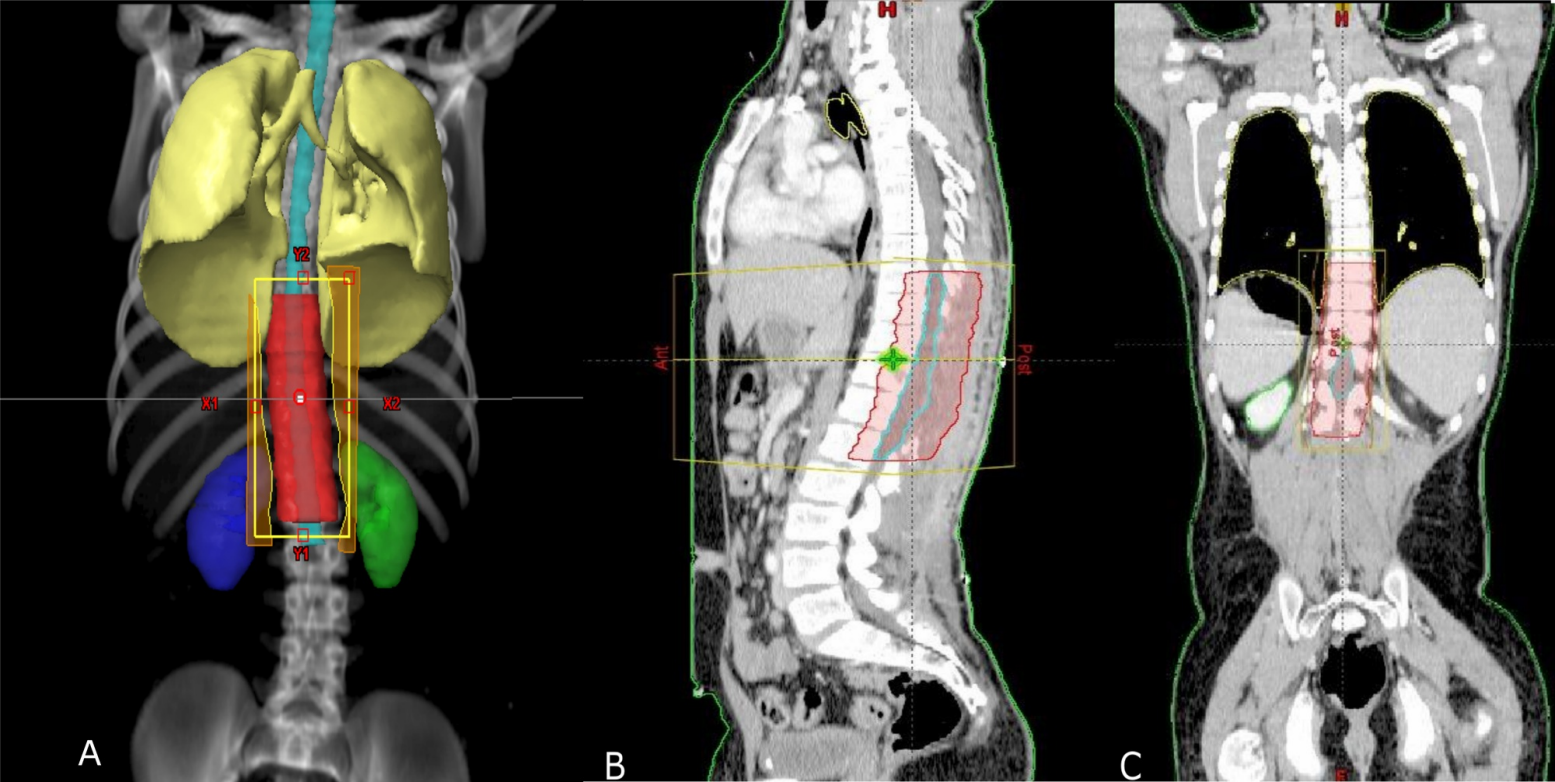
Radiation treatment plan. (A) Three-dimensional conformal radiation treatment plan showing digitally reconstructed radiograph. (B) Sagittal and (C) coronal views showing primary target volume in red.
